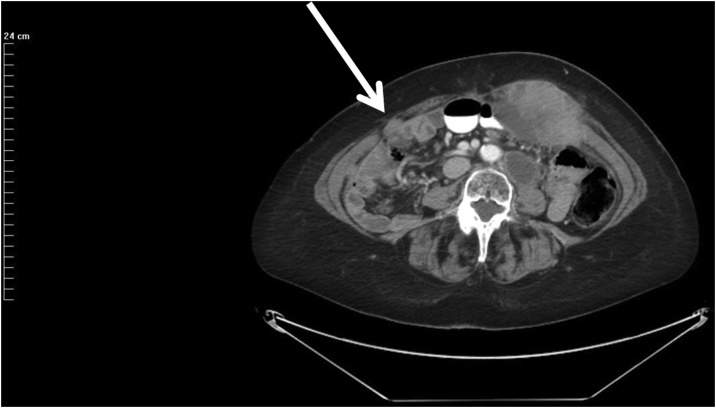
CT cut through the right mid-abdominal laparoscopy port site metastases. The anatomic location is through the fascia at the origin of the anterior and posterior rectus sheath. Although the left and right port site were tumor cell contaminated at the same time there is a marked size discrepancy. Progression of a left para-aortic lymph node is seen on this CT cut.
